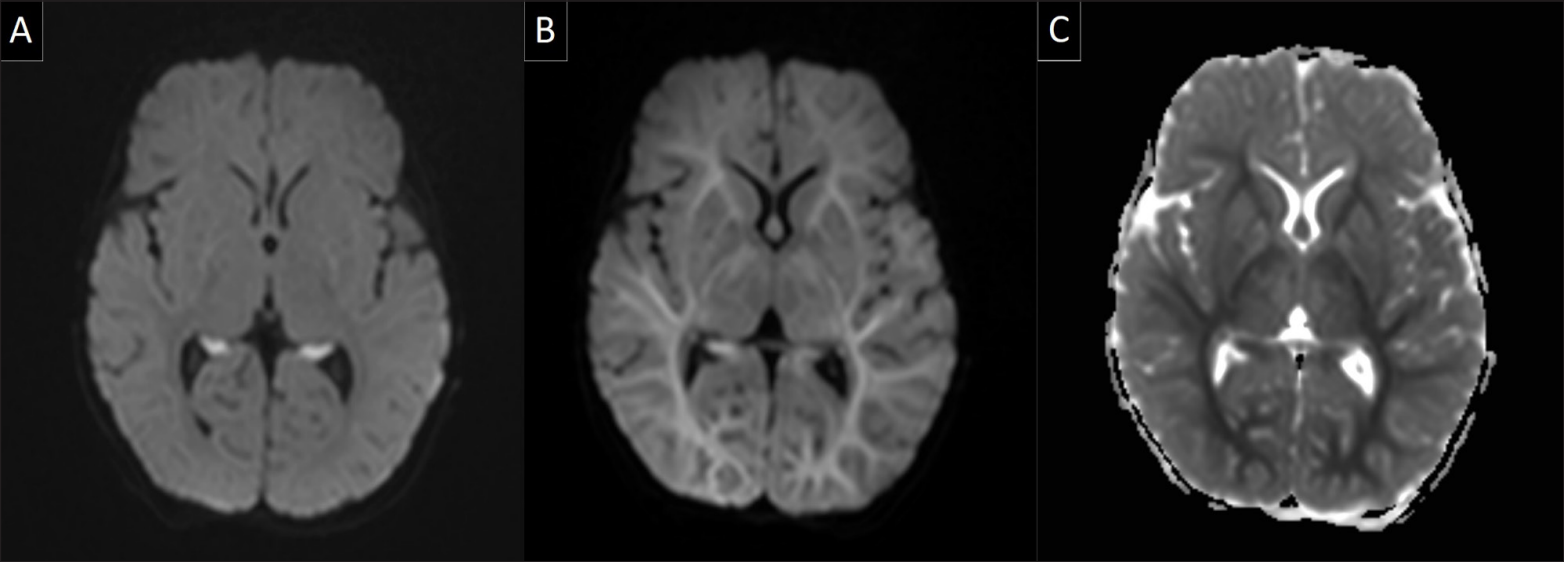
A. Axial diffusion weighted MR image (B1000) of the brain 1 day after the hypoxic-ischaemic event occurred shows extensive restricted diffusion in both hippocampi. There is no white matter involvement visible. B. Axial diffusion weighted MR image (B1000) of the brain 3 days after the hypoxic-ischaemic event occurred shows delayed post anoxic white matter injury with the white matter reversal sign: there is new extensive and symmetric diffuse high signal in the superficial and deep white matter, with relative sparing of the frontal white matter. C. Axial ADC image shows low ADC values in the affected white matter, confirming the restricted diffusion.
